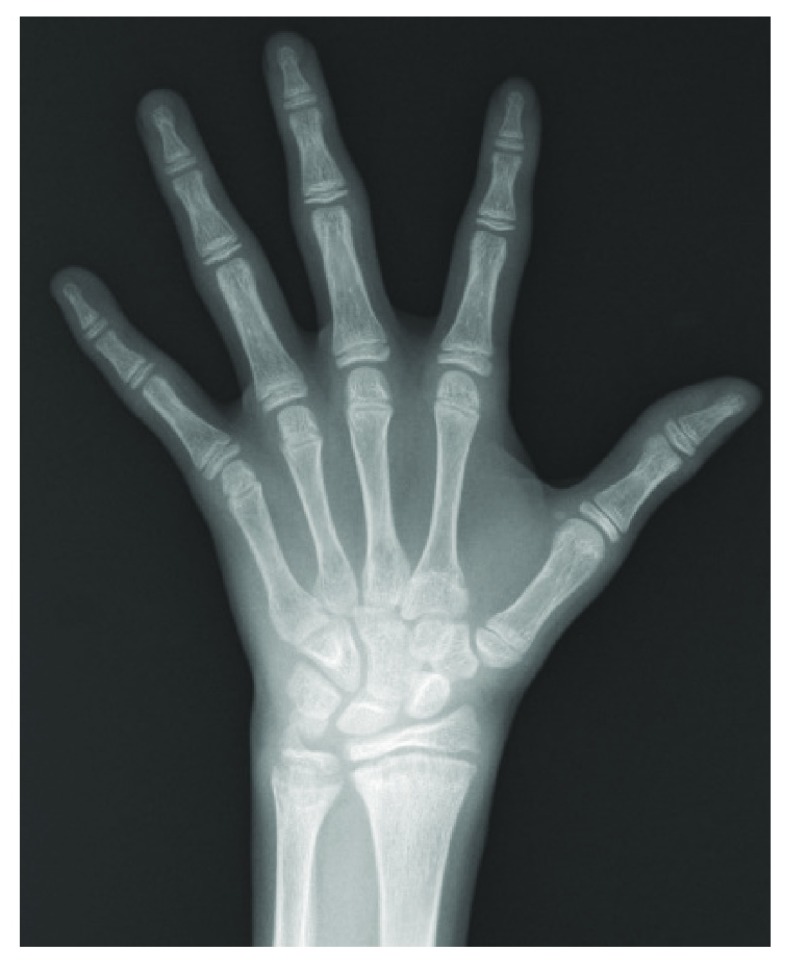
X-ray of the left hand of the patient with hypokalemic periodic paralysis. It showed a bone at the age of 13 years and 6 months.
